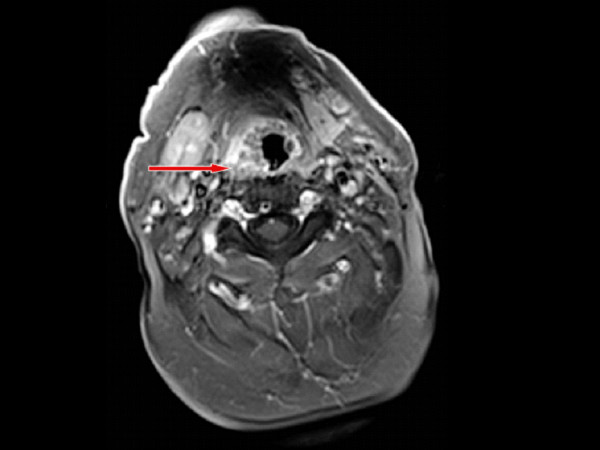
Axial intermediate T1 Fat saturated MRI, post gadolinium on lesion on right base of tongue. Lesion has irregular enhancement following gadolinium
